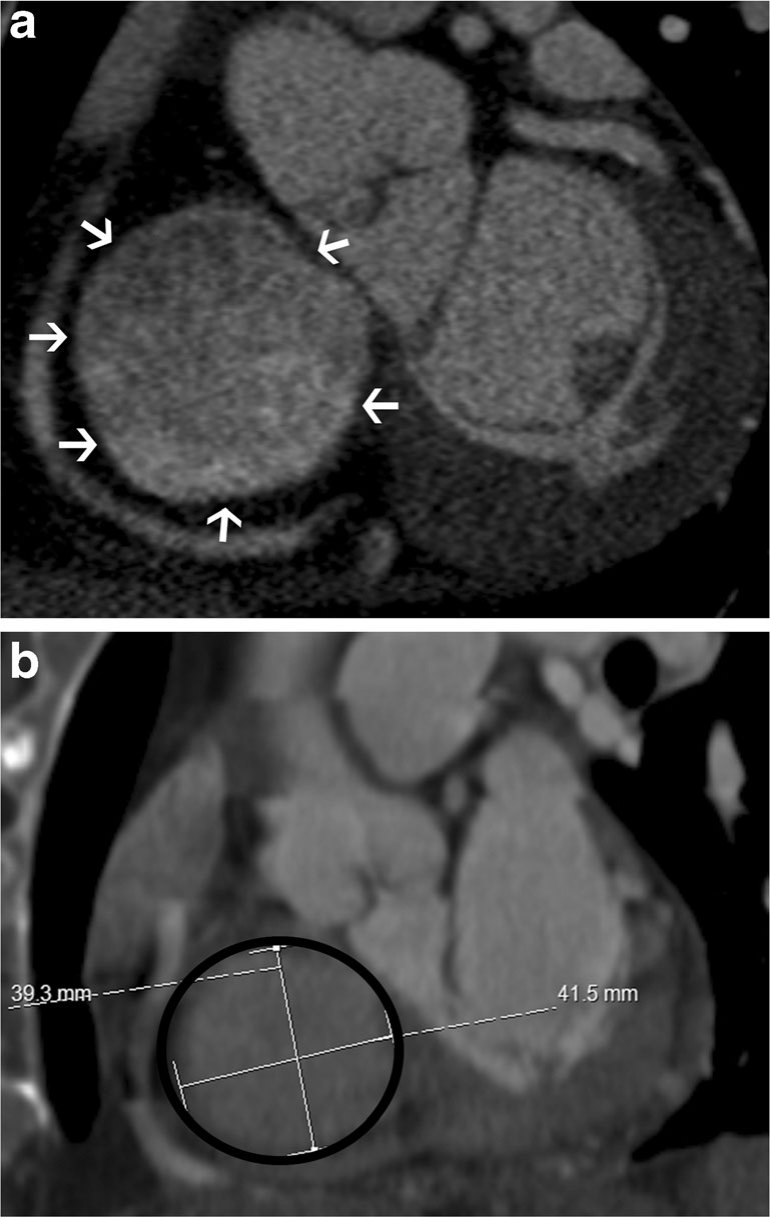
Tricuspid annulus. (a) Short-axis CT image of the tricuspid annulus,which is oval shaped and non-planar, with the septal leaflet attached in an oblique plane to the septum. The posterolateral is the lowest portion and the anteroseptal the highest portion of the annulus. (b) Measurement of the tricuspid annulus in CT, including circumference and diameters
